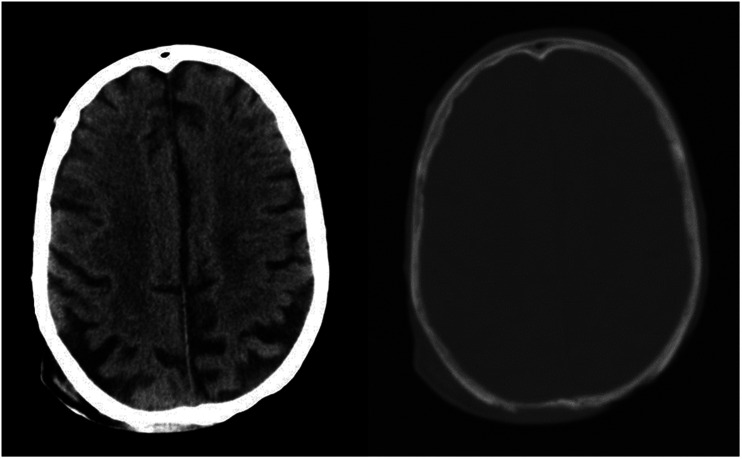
Contrast-enhanced CT head showing a large soft tissue defect over the right superior occipitoparietal area with marked thinning of the outer table of the underlying calvarium.
